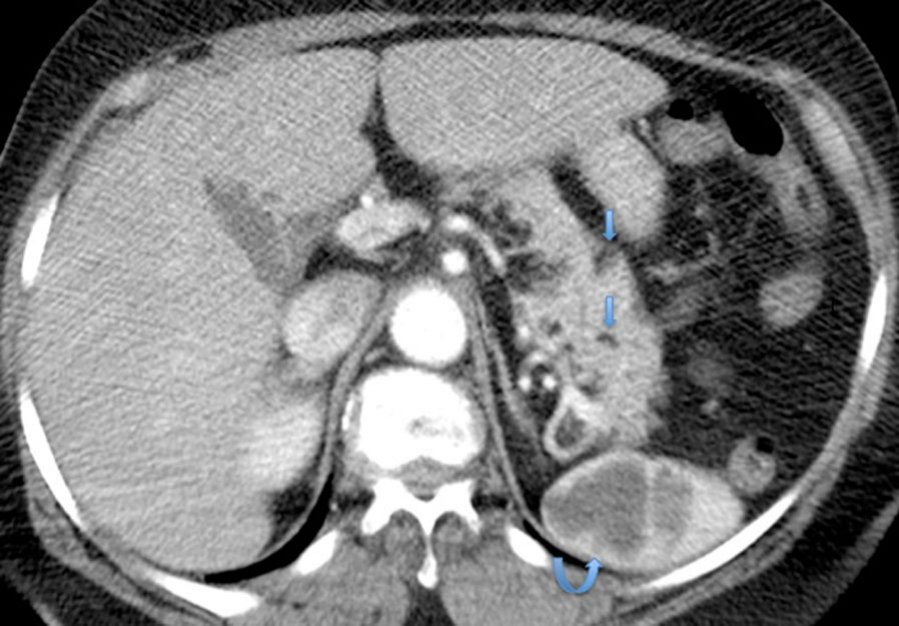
Axial contrast enhanced CT of the Abdomen. Multiple hypo enhancing splenic lesions were seen (*curved arrow*), likely representing hamartomas as well as subcentimeter fat density lesions within the pancreas (average HU = −70) (*straight arrows*)
